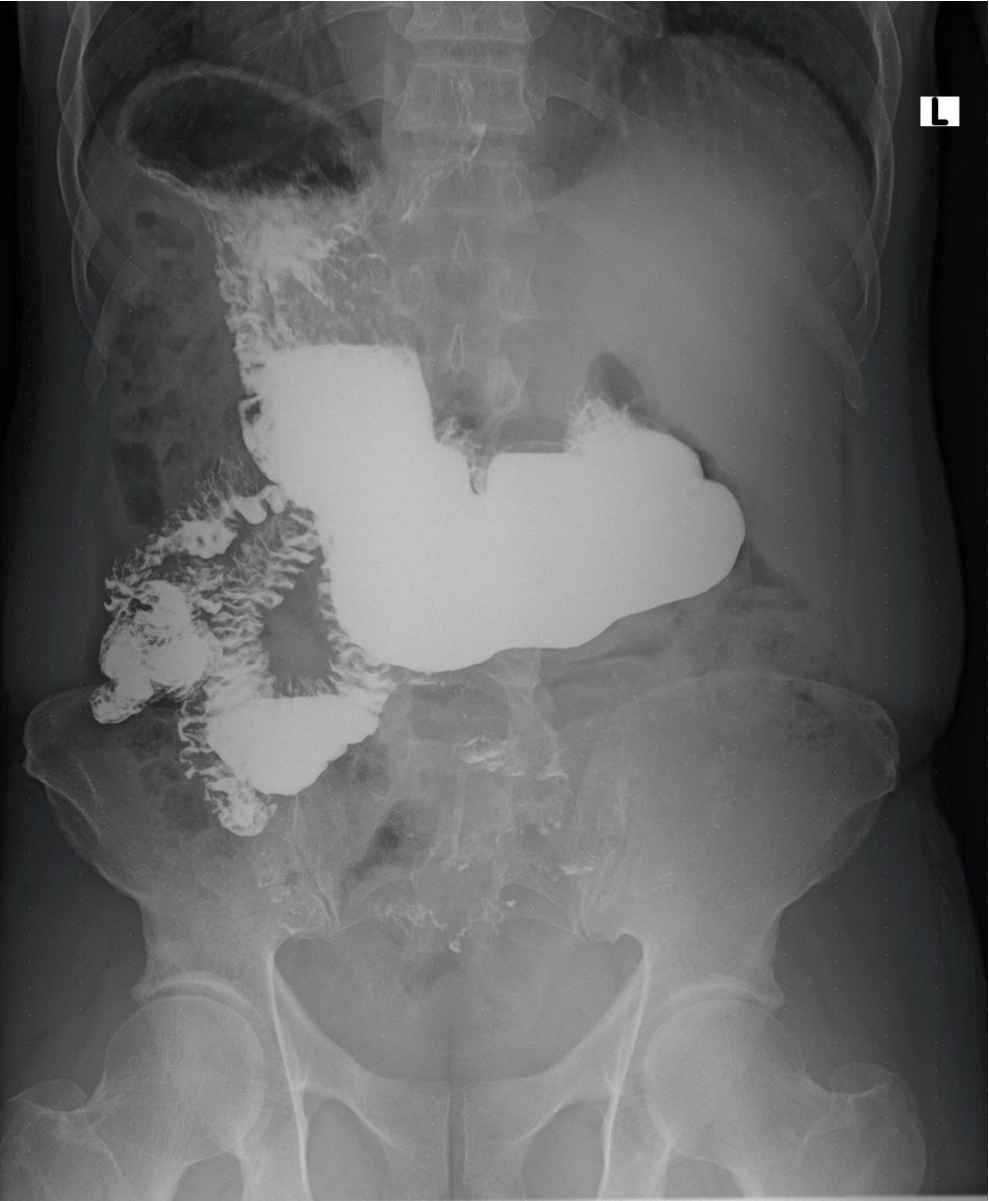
Barium sulphate meal spot radiograph. This shows the stomach on the right under the right hemidiaphragm, a definite air-barium level under the right hemidiaphragm with the heavier barium inferiorly and gastric air superiorly.
